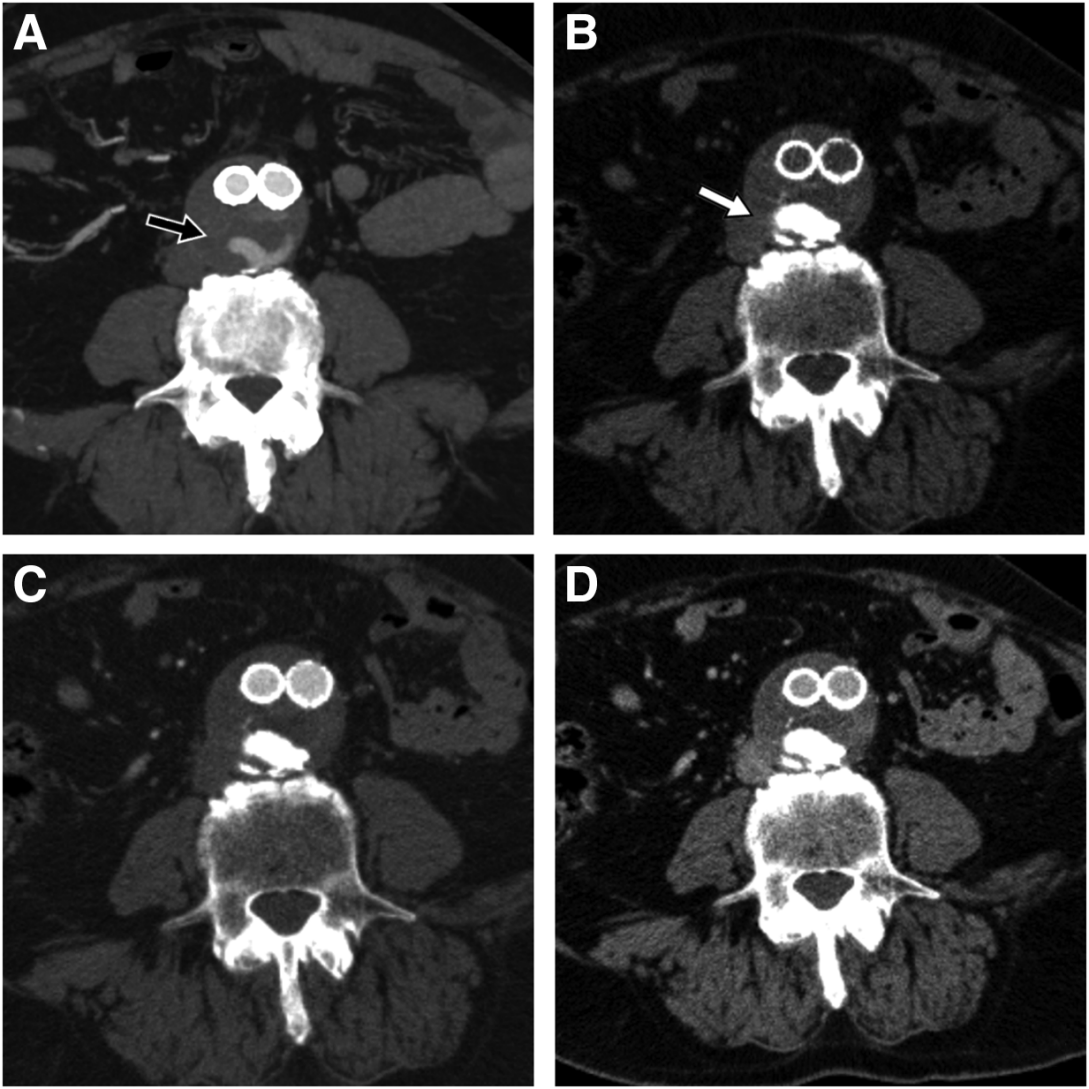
Pre-procedural CTA showing part of the endoleak during arterial phase (black arrow) (A), 6-month follow-up CTA on the same anatomic level showing easyx cast in unenhanced (white arrow) (B), arterial (C), and venous phase (D) with complete disappearance of the T2E.
